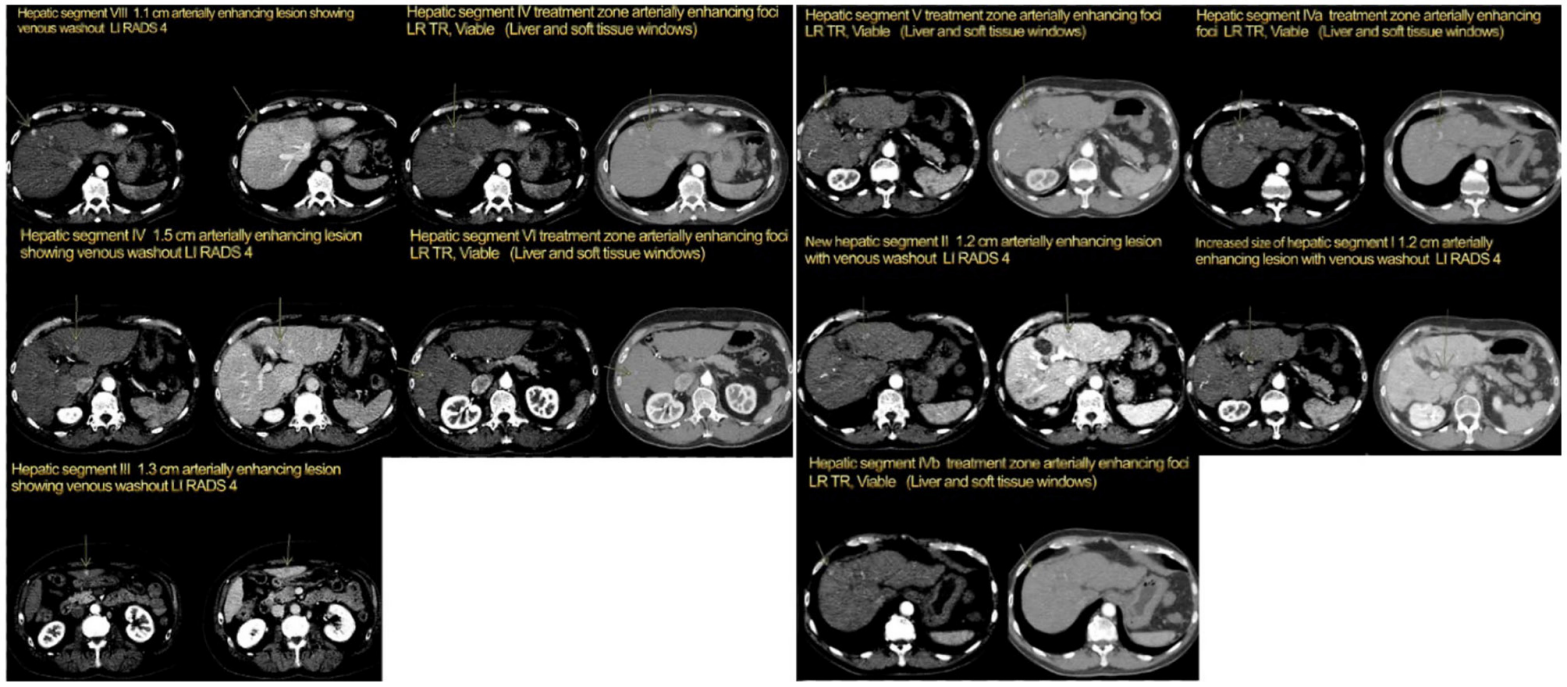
Patient 1, four-phase computed tomography (CT) scan: Left: pre-cabozantinib (July 2016), Right: post-cabozantinib/pre-transplant (August 2019).
